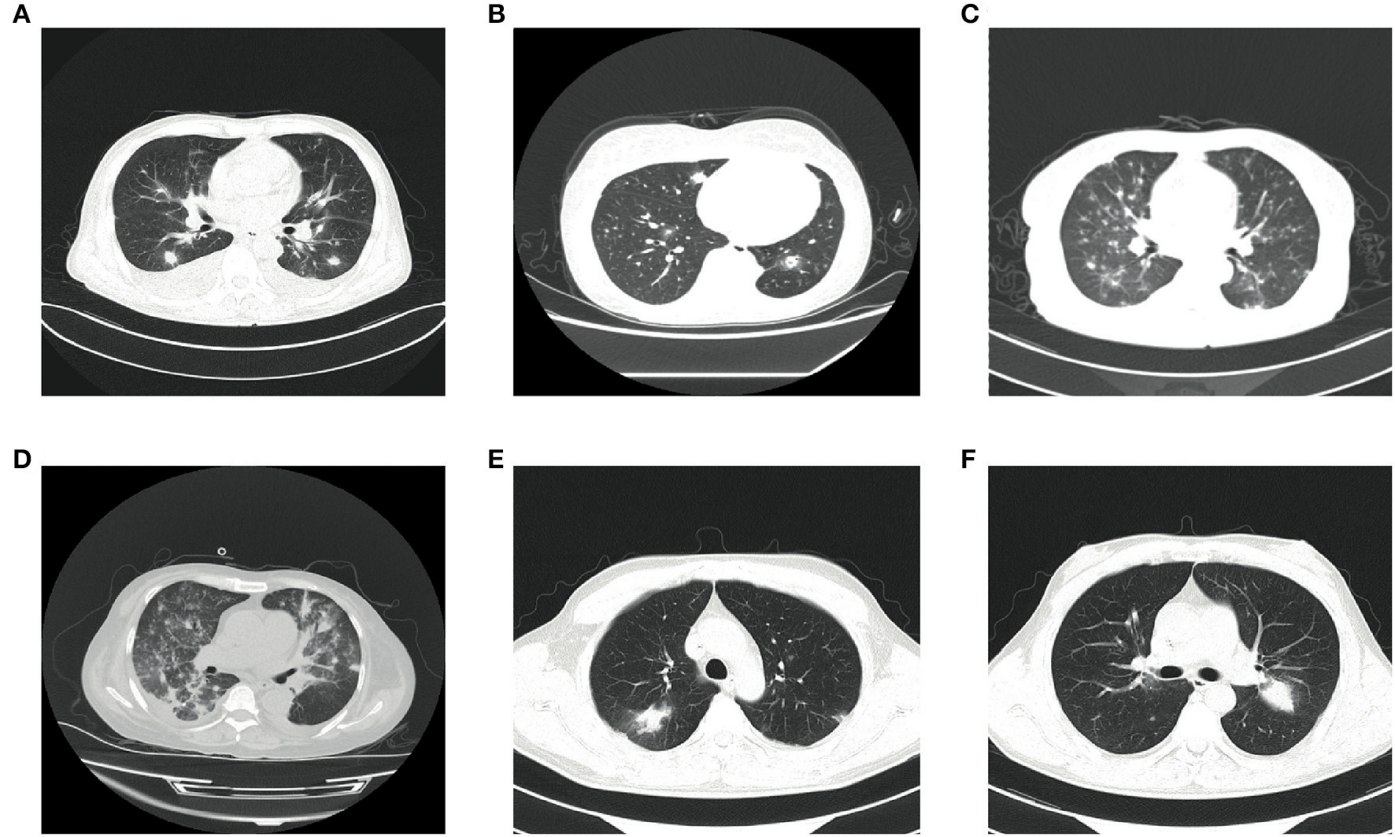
Findings of chest CT scan. (A) Chest CT of case 1 showing multiple small nodules in both lungs and a little pleural effusion on both sides. (B) Chest CT of case 2 showing multiple small nodules in both lungs and halo signs around some nodules. (C) Chest CT of case 3 showing multiple small nodules along the vascular bundles in both lungs and a little pleural effusion on both sides. (D) Chest CT of case 4 showing multiple irregular nodules in both lungs and a little pleural effusion on the right side. (E,F) Chest CT of case 5 showing multiple nodules and masses in both lungs.
